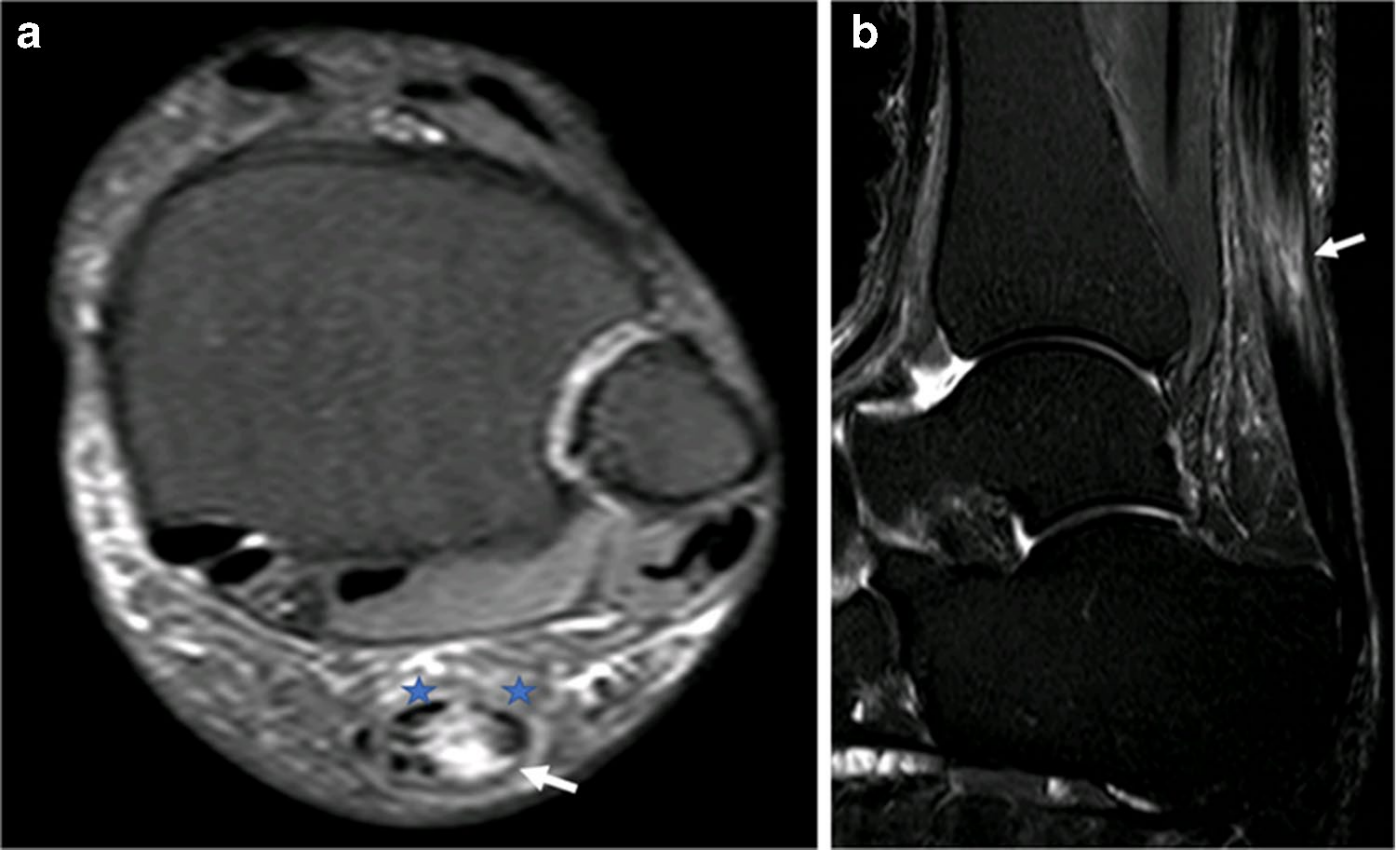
MRI of the left ankle in a 51-year-old woman who experienced acute onset severe pain in the distal calf 4 days after an ultrasound-guided peritendinous Achilles corticosteroid injection. Axial (a) and sagittal (b) STIR images of the left ankle demonstrate near complete rupture of the Achilles tendon (arrows) at the musculotendinous junction. Some peripheral fibres remain intact preventing total discontinuity (stars in (a))
